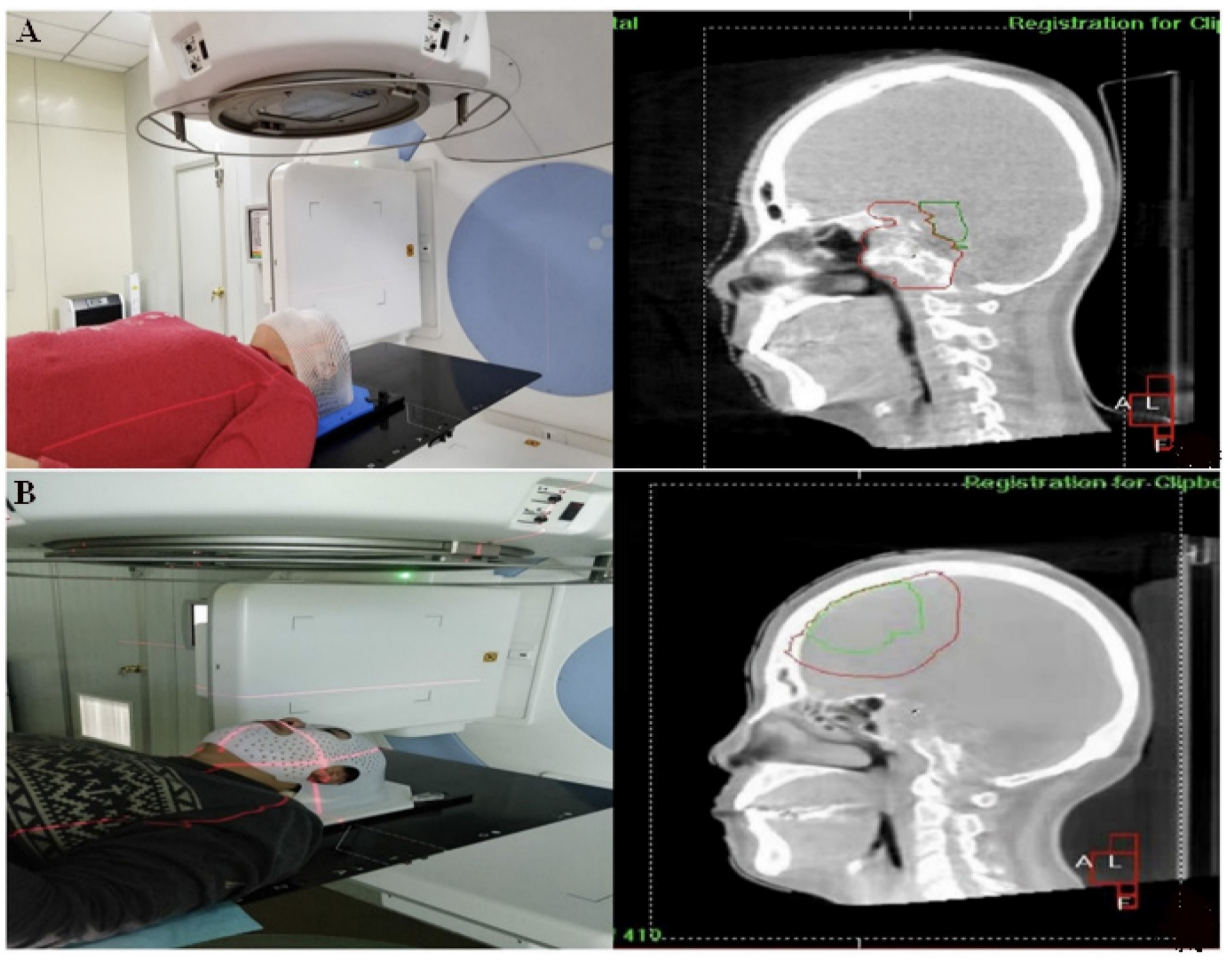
Patients treated with the thermoplastic head membrane plate and kV-CBCT images (A) and patients treated with 3D printing head membrane and kV-CBCT images (B).
